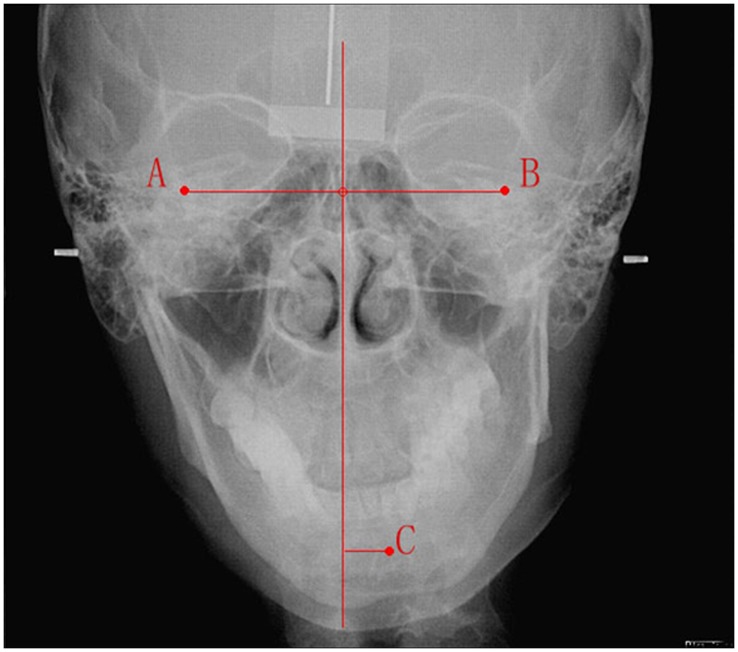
Anterior X-ray film of head. The midpoints of the inferior orbital fissures were designated as points A and B, and the midpoint of the chin ridge was as point C.
